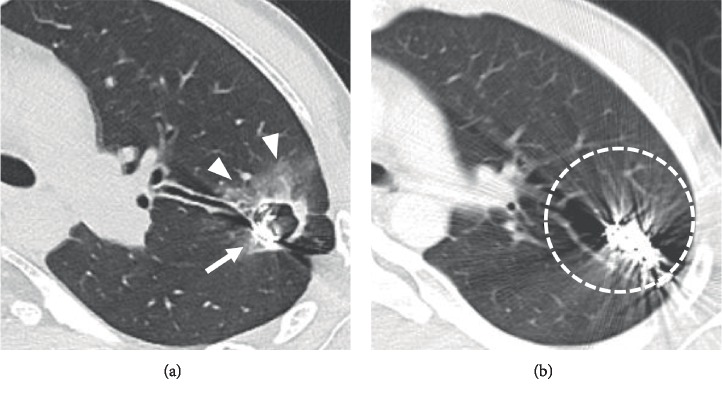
(a) CT shows a cavity lesion including the coils in the left upper lobe (arrow) and ground-glass opacity around the cavity lesion (arrow heads). We suspected that the ground-glass opacity might represent the source of the bleeding. (b) CT performed 10 years earlier shows no cavity lesions.
